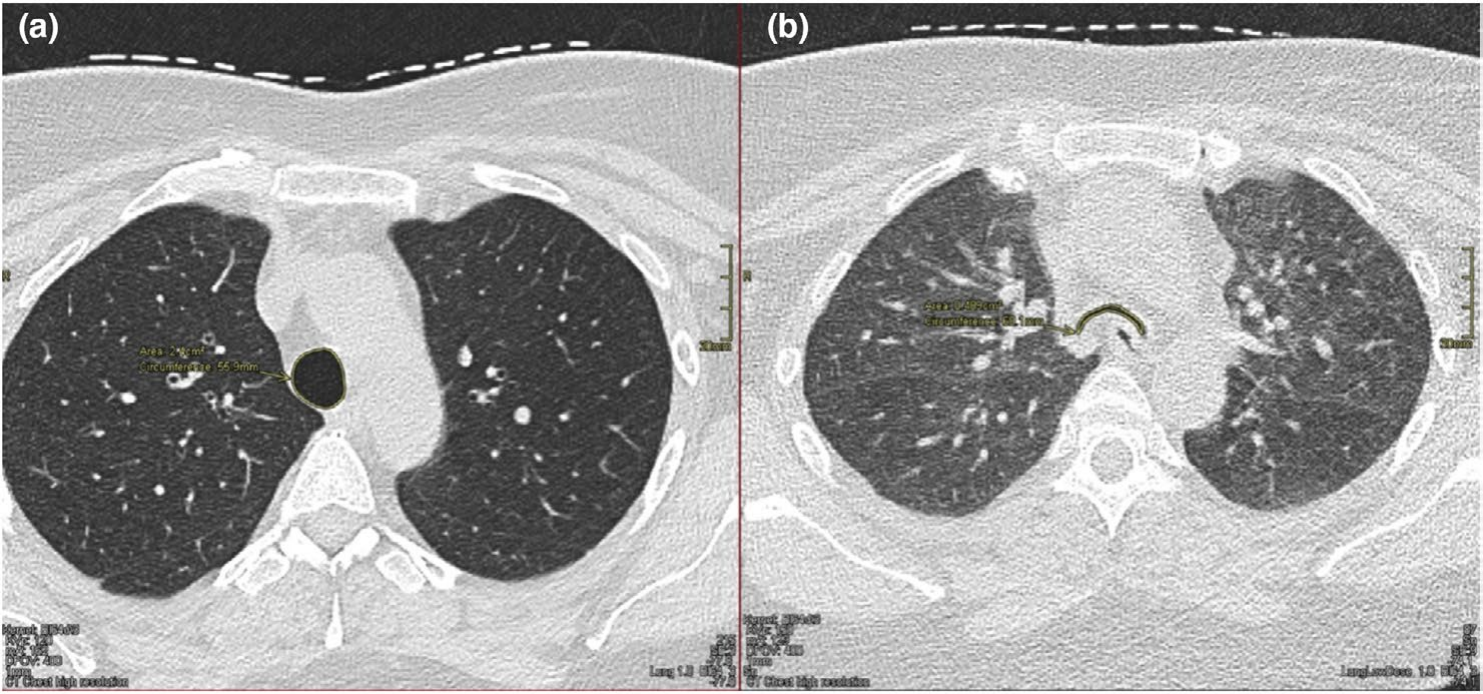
Axial CT imaging of a 53‐year‐old female. (a) End inspiratory CT image of the trachea displaying a round, circular shape, with a cross‐sectional area of 2.8 cm^2^. (b) End expiratory image at a similar tracheal level displaying a bowing, crescent shape flattening of the posterior membrane. Cross‐sectional area decreased to 0.41 cm^2^, an 85% reduction.
